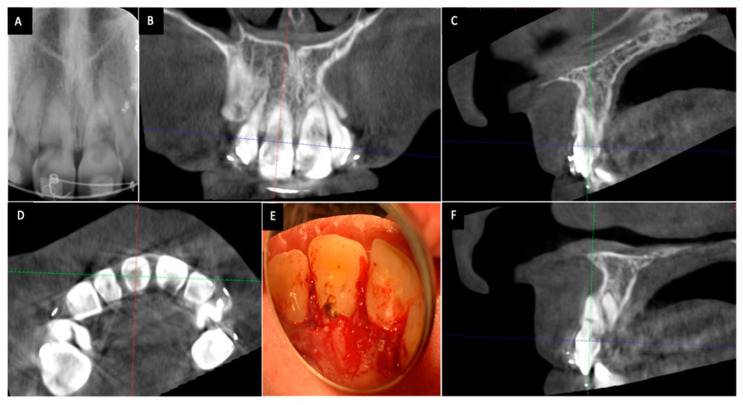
Cone-beam computed tomography (CBCT) shows a more accurate assessment of invasive cervical resorption (ICR) lesions than two-dimensional radiographs. The size and locations of ICR lesions can be determined with CBCT. (A) Periapical radiograph showing ICR lesions in maxillary right and left incisors; (B) The coronal view of CBCT scan image showing ICR lesions in maxillary right and left incisors;(C) The sagittal view of CBCT scan image (maxillary right incisor); (D) The axial view of CBCT scan image showing the ICR lesion in maxillary right and left incisors; (E) Clinical view of ICR lesions located on the palatal surface of the teeth; (F) The sagittal view of CBCT scan image showing the ICR lesion in maxillary left incisor.
